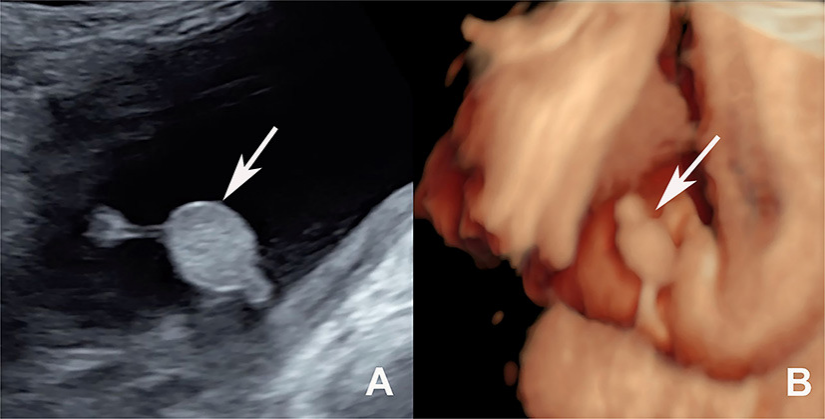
(A) Two-dimensional ultrasound showed a hyperechoic mass resembling a scrotum (the white arrow shows) in the perineum of the fetus. (B) Three-dimensional ultrasound showed the scrotum-like masses (the white arrow shows) and their relationship with other organs vividly at 33 weeks gestation.
